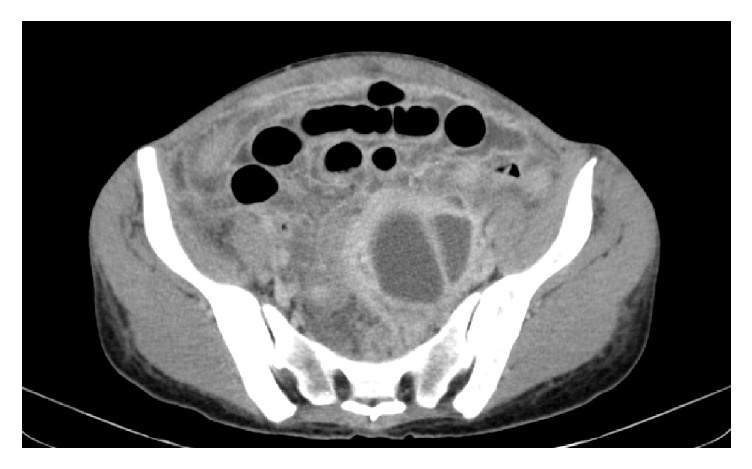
CT of pelvis demonstrating multiloculated abscess and dilated bowel loops.
